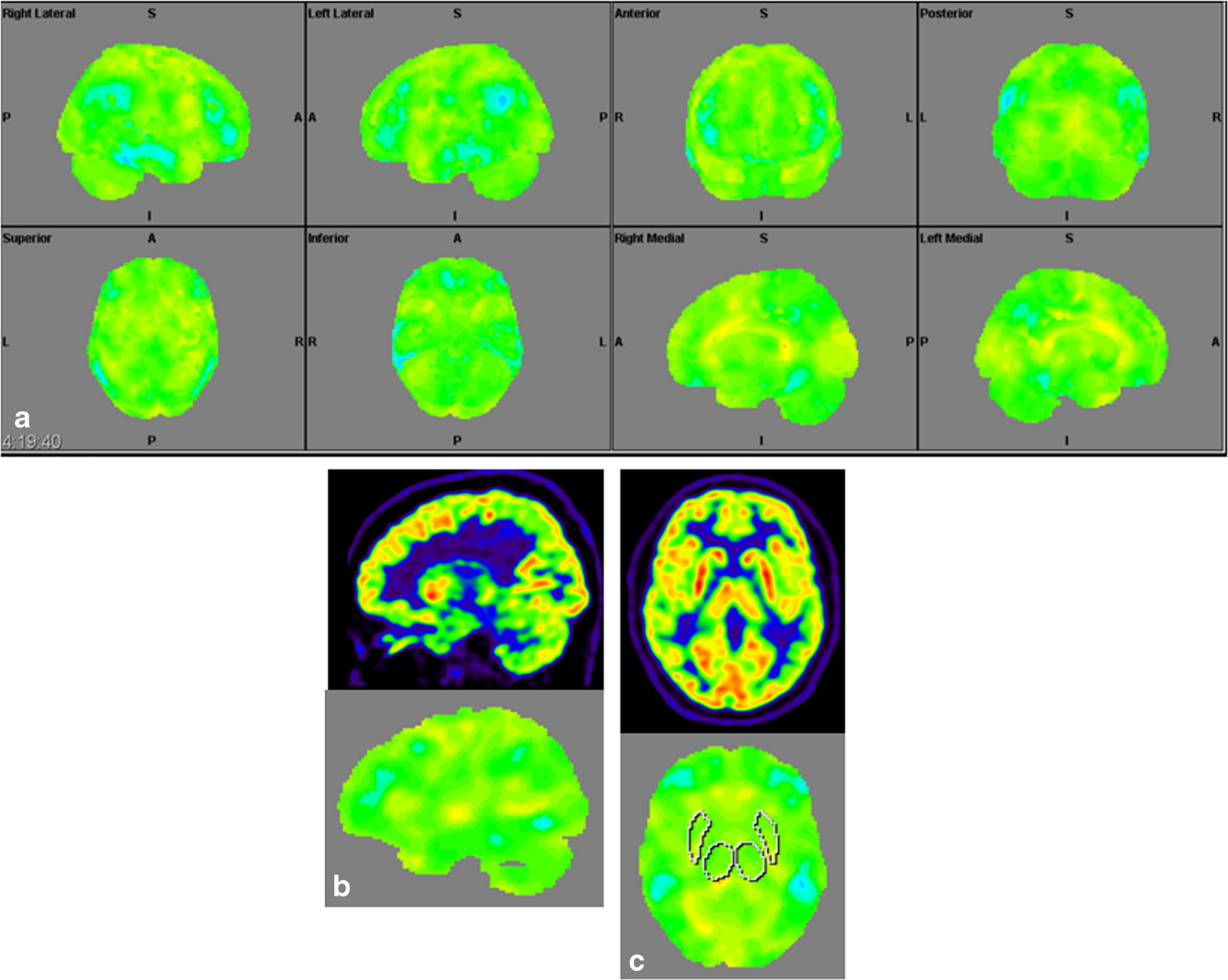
Surface projections (3DSSP) of brain [^18^F] FDG PET findings for patient V:1. a Displays hypometabolism in the prefrontal cortex, lateral temporal cortex, and in some areas of the parietal cortex. b Metabolism in the cerebellum is normal on this midsagittal image (upper case). c FDG uptake is also normal in the basal ganglia and thalami on the coronal image (upper case); reference values in VOI templates are displayed in the lower cases of B and C
